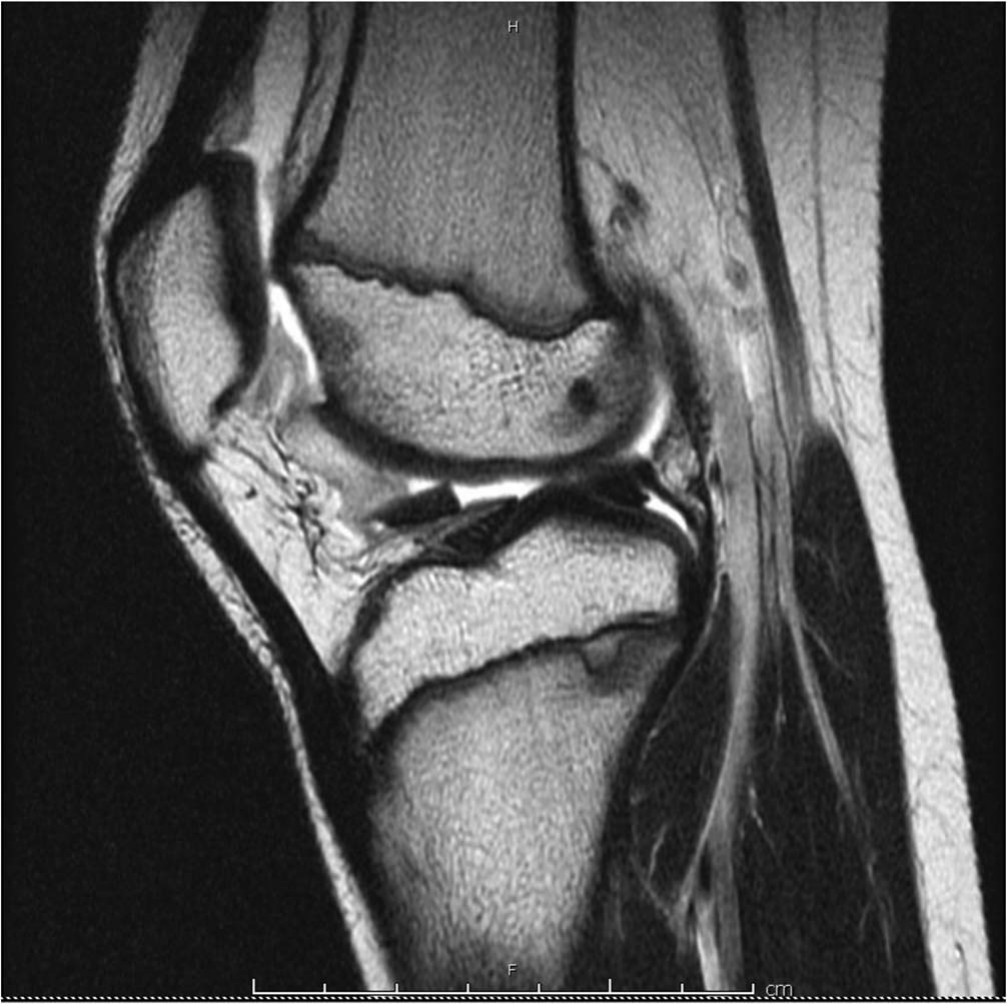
T1-weighted MRI showing full-thickness cartilage defect at the lateral femoral condyle.
